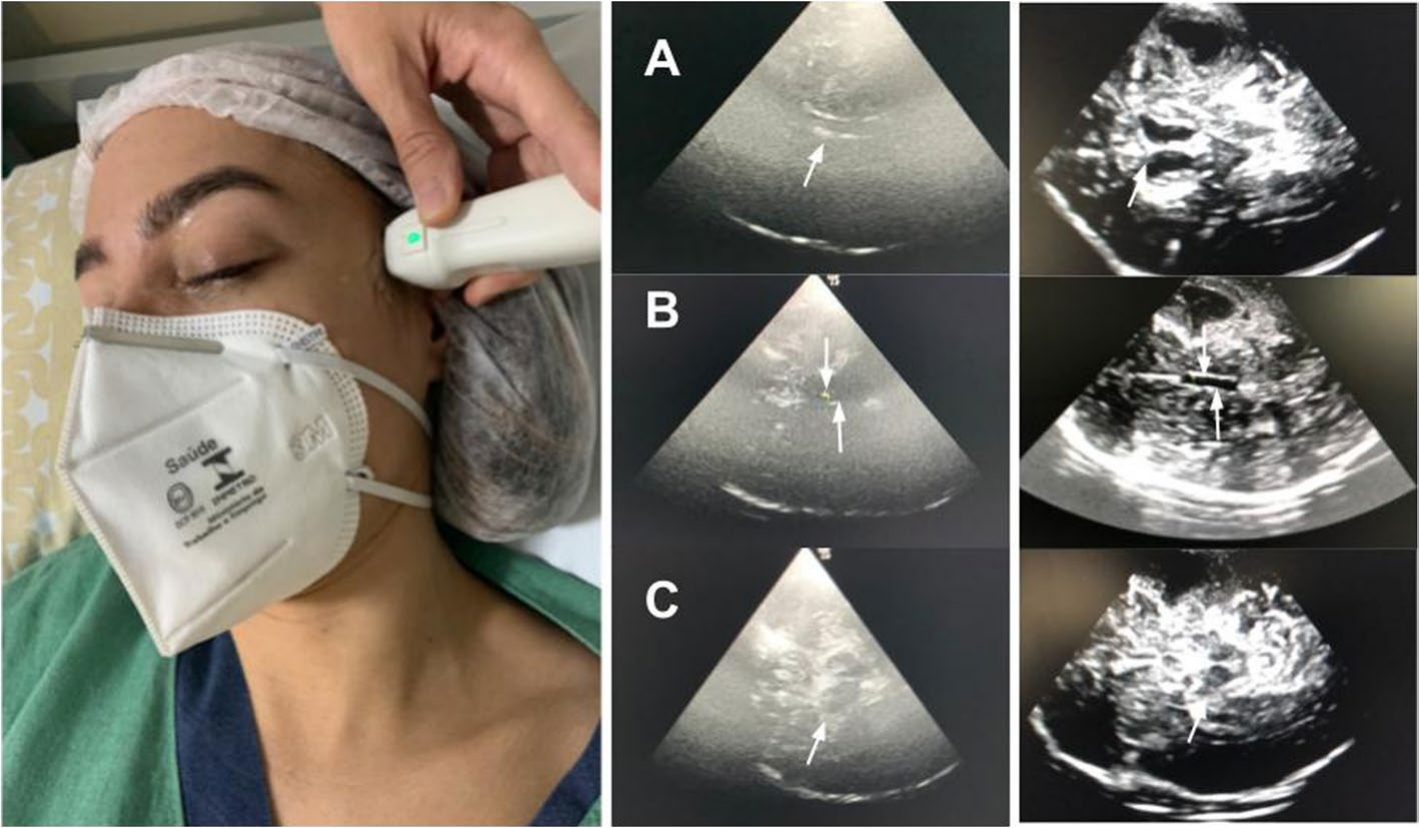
The transtemporal window approach and the three images of the brain’s ultrasonography planes. A Ventricular plan. B Diencephalic plan. C Mesencephalic plan
